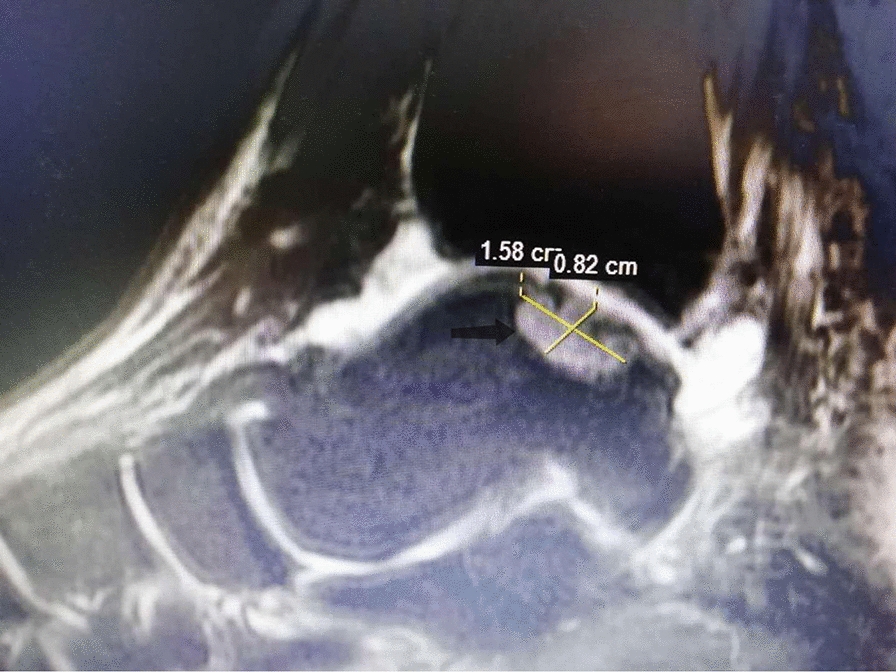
Ankle MRI of the 18-year-old young male patient. T2-weighted sagittal view of the ankle MRI revealed a 0.94 × 0.82 × 1.58 cm, well-defined, cystic structure in medial, central talus (arrow)
